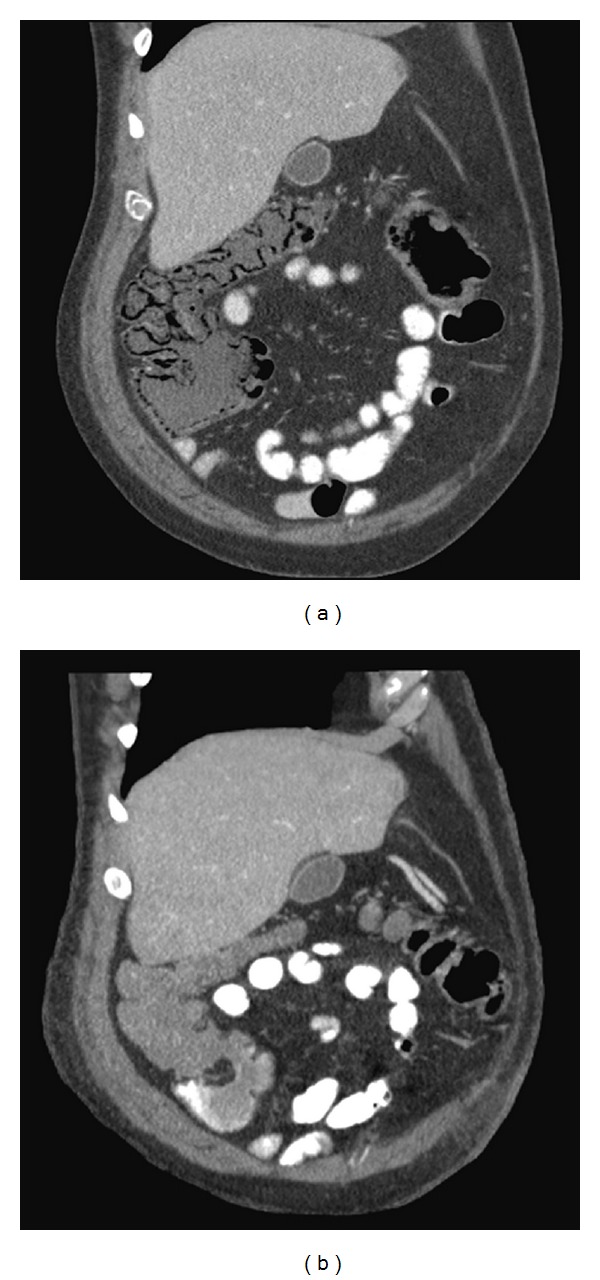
(a) CT scan of the abdomen 2 days following high-dose intravenous steroids shows extensive PI of right colon. Intramural gas tracking is visualized parallel to the bowel mucosa in the ascending and transverse colon. (b) Repeat CT scan after 3 days bowel rest and tapering of steroids and shows resolution of PI. No evidence of intramural gas along the colonic mucosa is seen.
